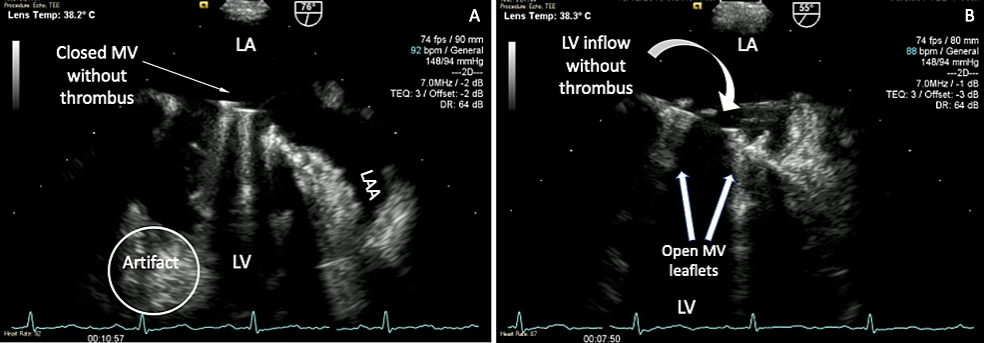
Transesophageal echocardiogram demonstrating thrombi resolution after thrombolysis with ultraslow high-dose t-PA Transesophageal echocardiogram showing (A) closed prosthetic mechanical mitral valve with resolution of the previously seen sessile thrombi (one arrow). (B) LV inflow with prosthetic mechanical mitral valve strands (curved arrow) and open prosthetic mitral valve leaflets (two arrows) without the previously seen large mobile thrombus. LA = left atrium, LAA = left atrial appendage, LV = left ventricle, MV = mitral valve
